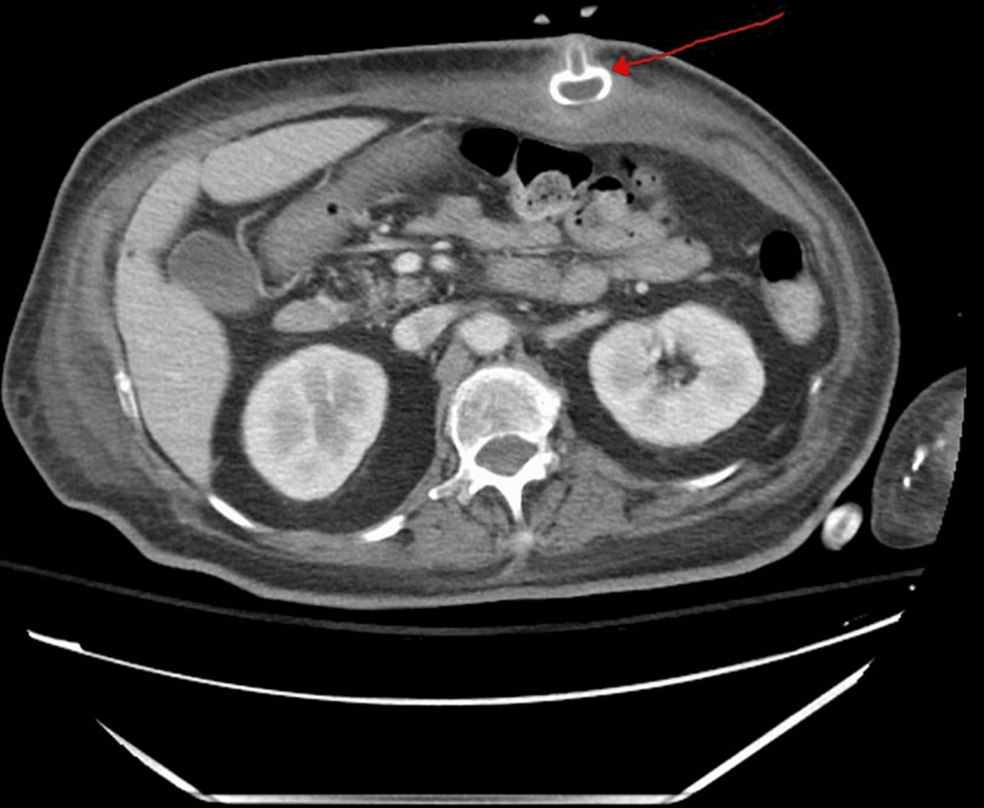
Computerized tomography scan of the abdomen and pelvis with contrast showing the PEG tube internal bolster buried in the gastric wall. An axial view of the CT scan of the abdomen and pelvis demonstrating the displacement of the PEG tube internal bolster into the gastric wall. The internal bumper appears extrinsic to the stomach, impinging on the gastric wall but not within the lumen.
